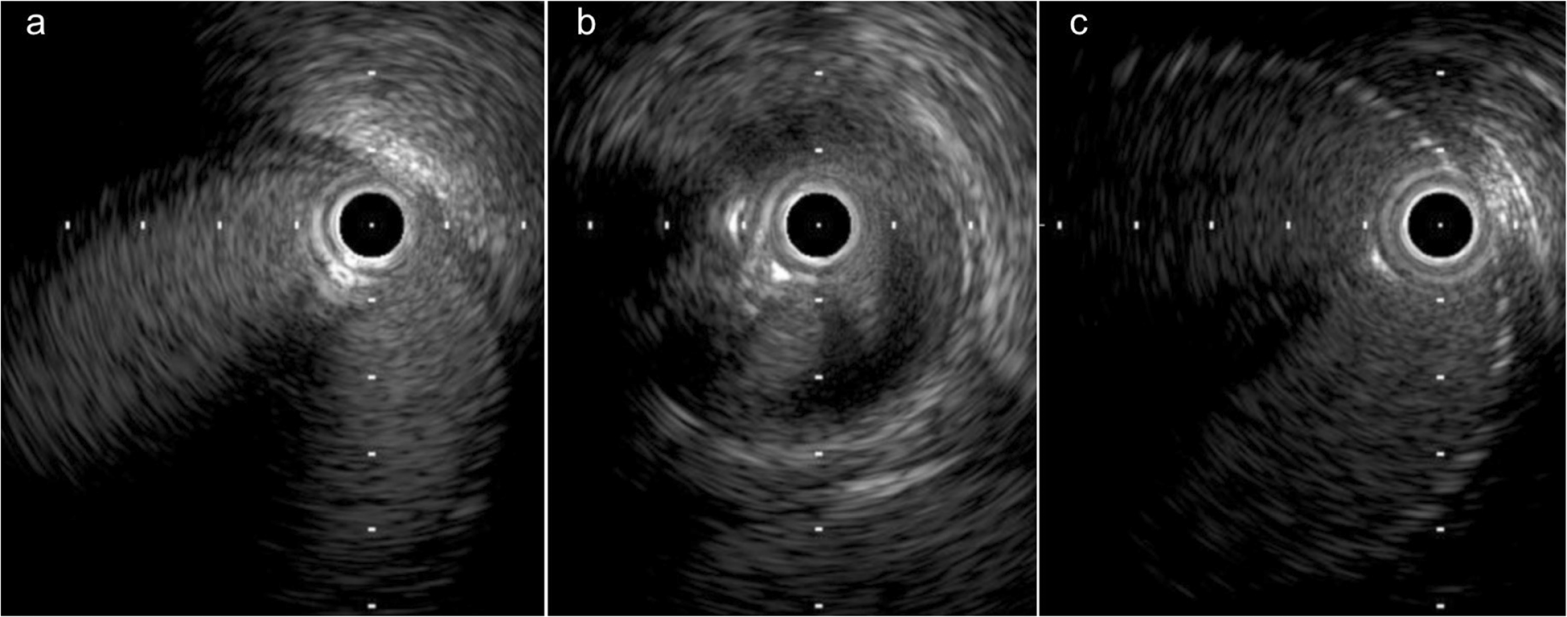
Intravascular ultrasonography showing vessel shrinkage at the narrowing part, with a diameter of 5 mm (a proximal part; b the narrowing part; c distal part)
